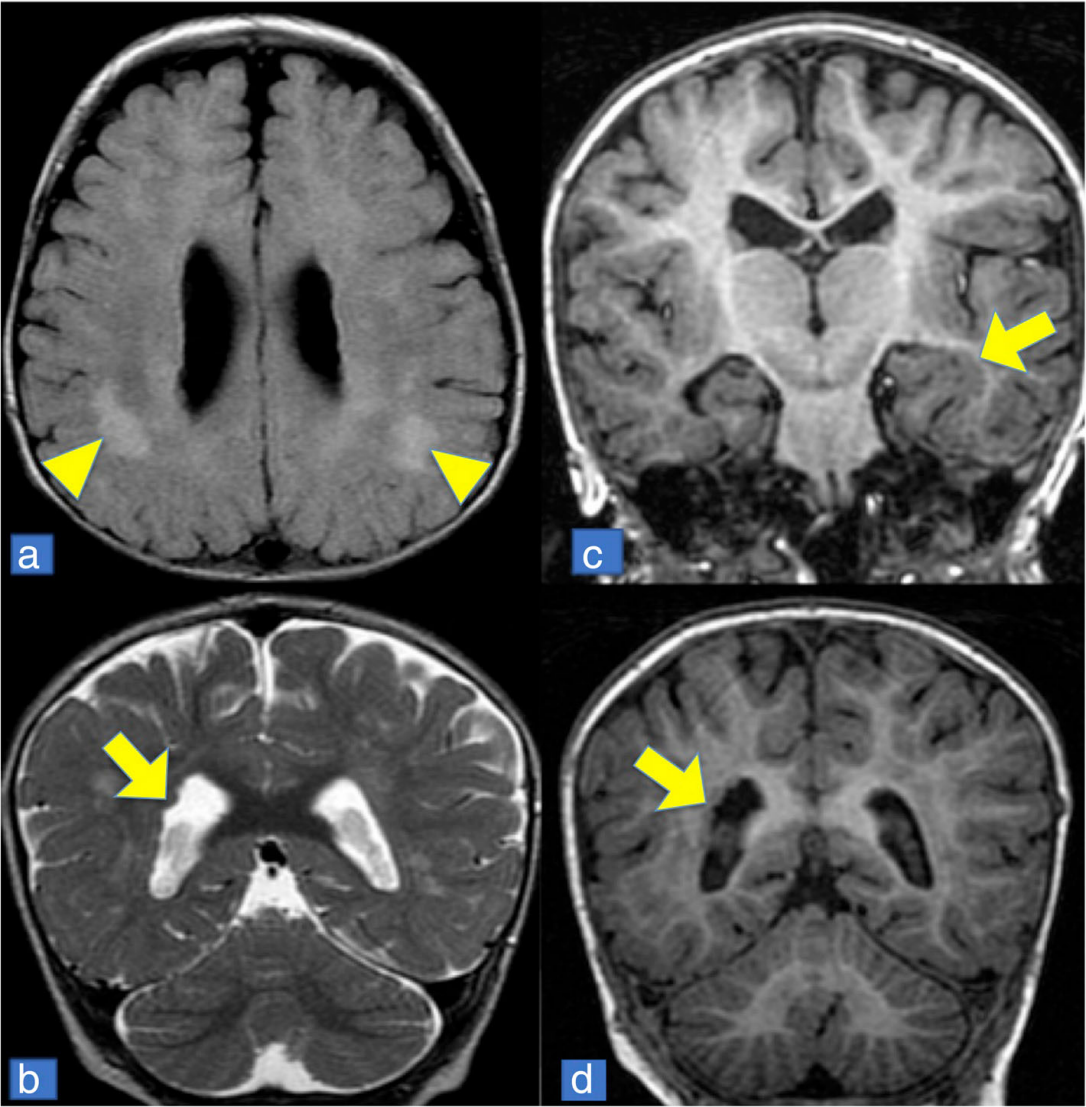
Brain MRI. Appearance of nodules (indicated by arrows) in periventricular grey matter heterotopia (images b, e, d), surrounding the left temporal horn and merging with the hippocampal cortex (image c). Supratentorial signal alterations with T2 and FLAIR hyperintense (images a, indicated by triangles) as in demyelinating lesions
